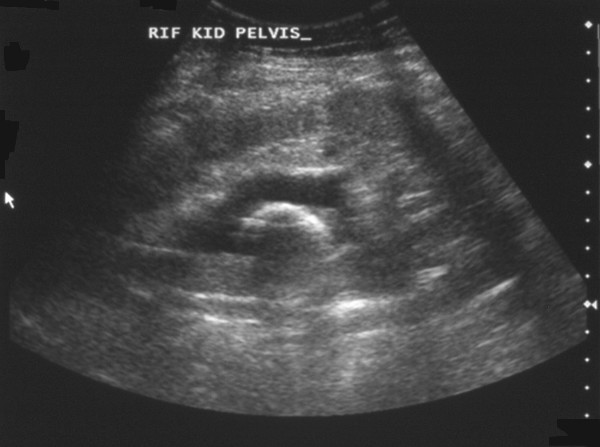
Ultrasound scan demonstrating hyper-reflective encrusted ureteric stent.
